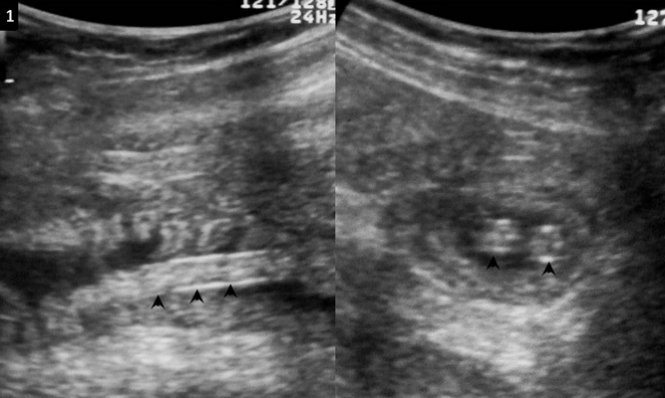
Ultrasound image of small bowel showing *Ascaris* in longitudinal and transverse views.
